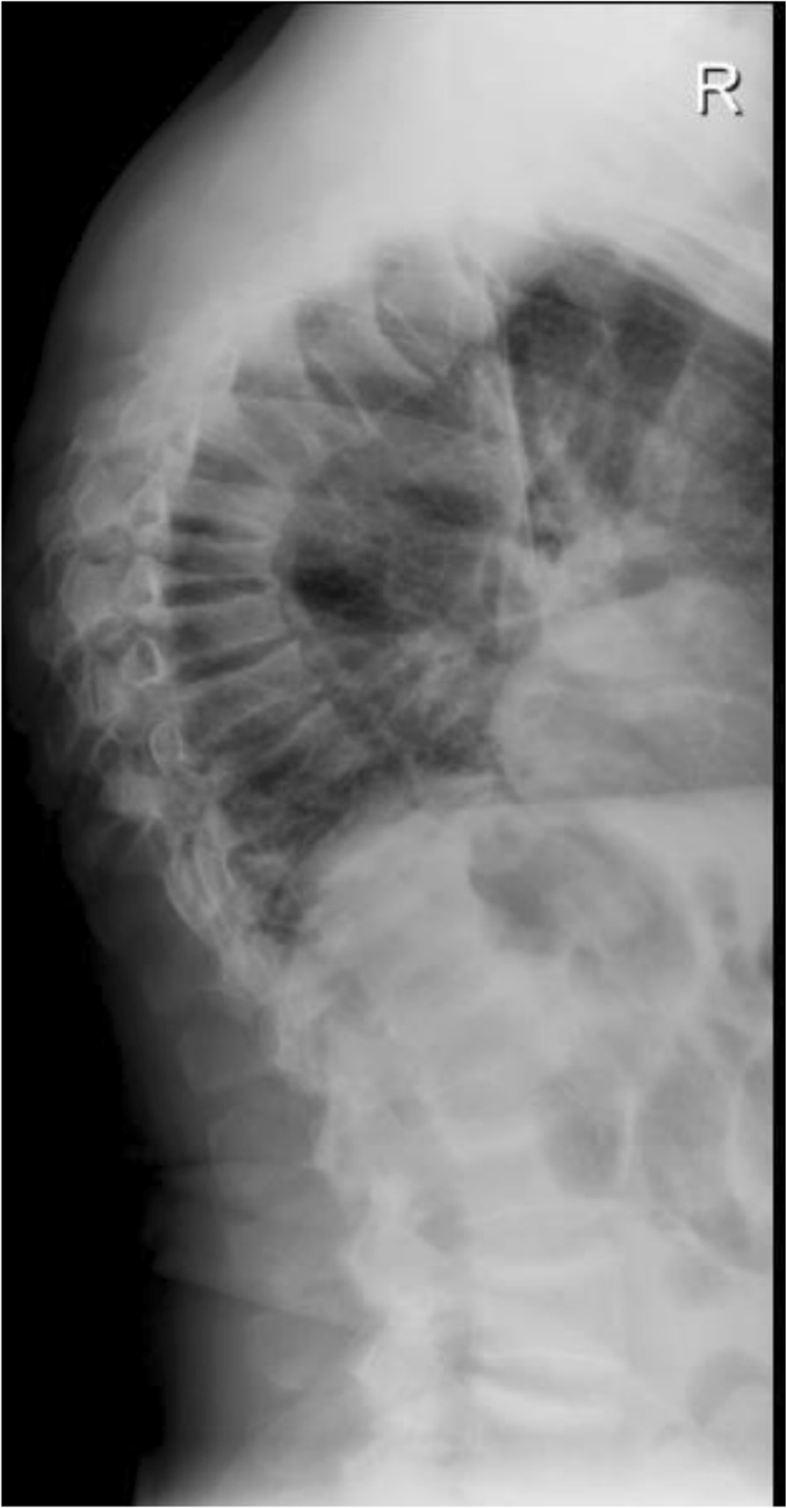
Conventional radiography of the spine after 1 year after tumour resection showed permanent and nonreversible spine deformation due to the old compressions from Th3 to Th11 with no evidence of new compression fractures (year 2015)
